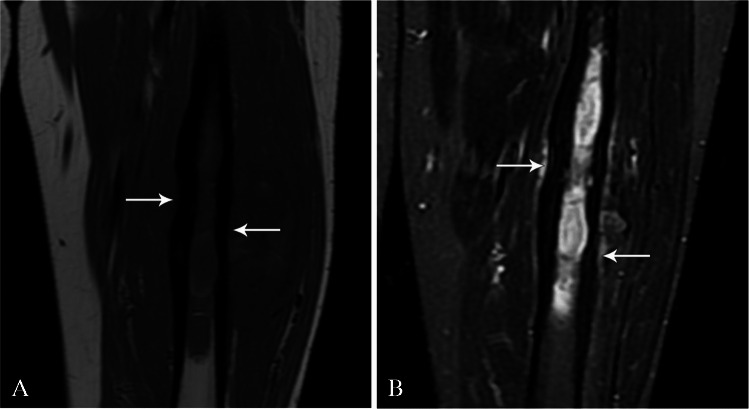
26-year-old male with Ewing sarcoma of the left mid femur. Coronal T1 (A) and STIR (B) MRI images demonstrate cortical thickening with saucerization defects (arrows) of both the medial and lateral aspects of the femoral shaft
